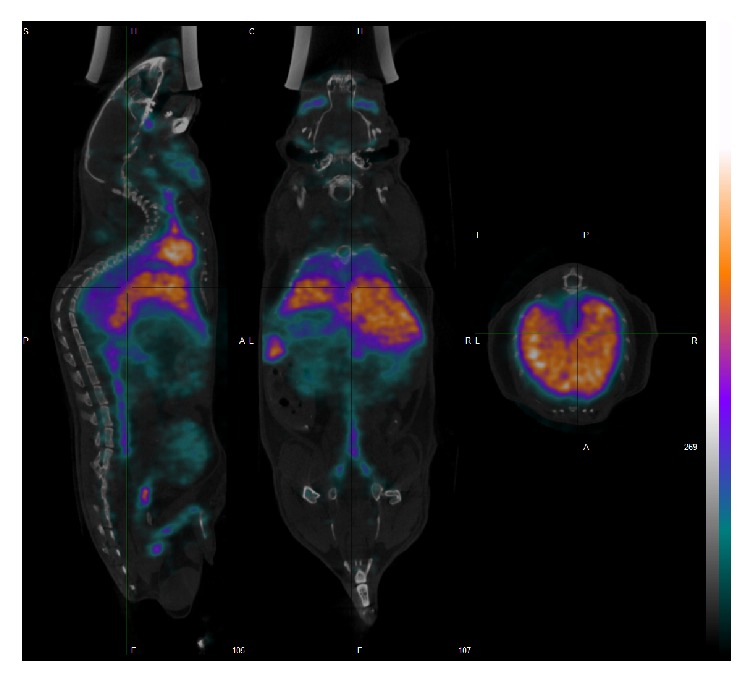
In vivo SPECT/CT images of the ^99m^Tc-labeled liposomes. The 3D reconstructed and coregistered SPECT and CT image are shown together with sagittal, coronal, and axial images (from left to right). Uptake of the liposomes by the liver and spleen is clearly visible in the images.
